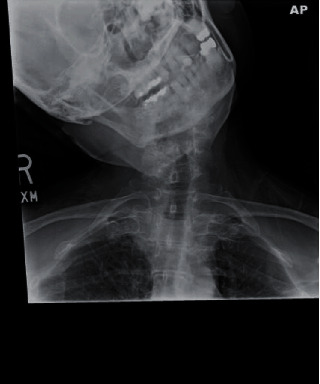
Preoperative cervical X-ray demonstrating severe right laterocollis.
